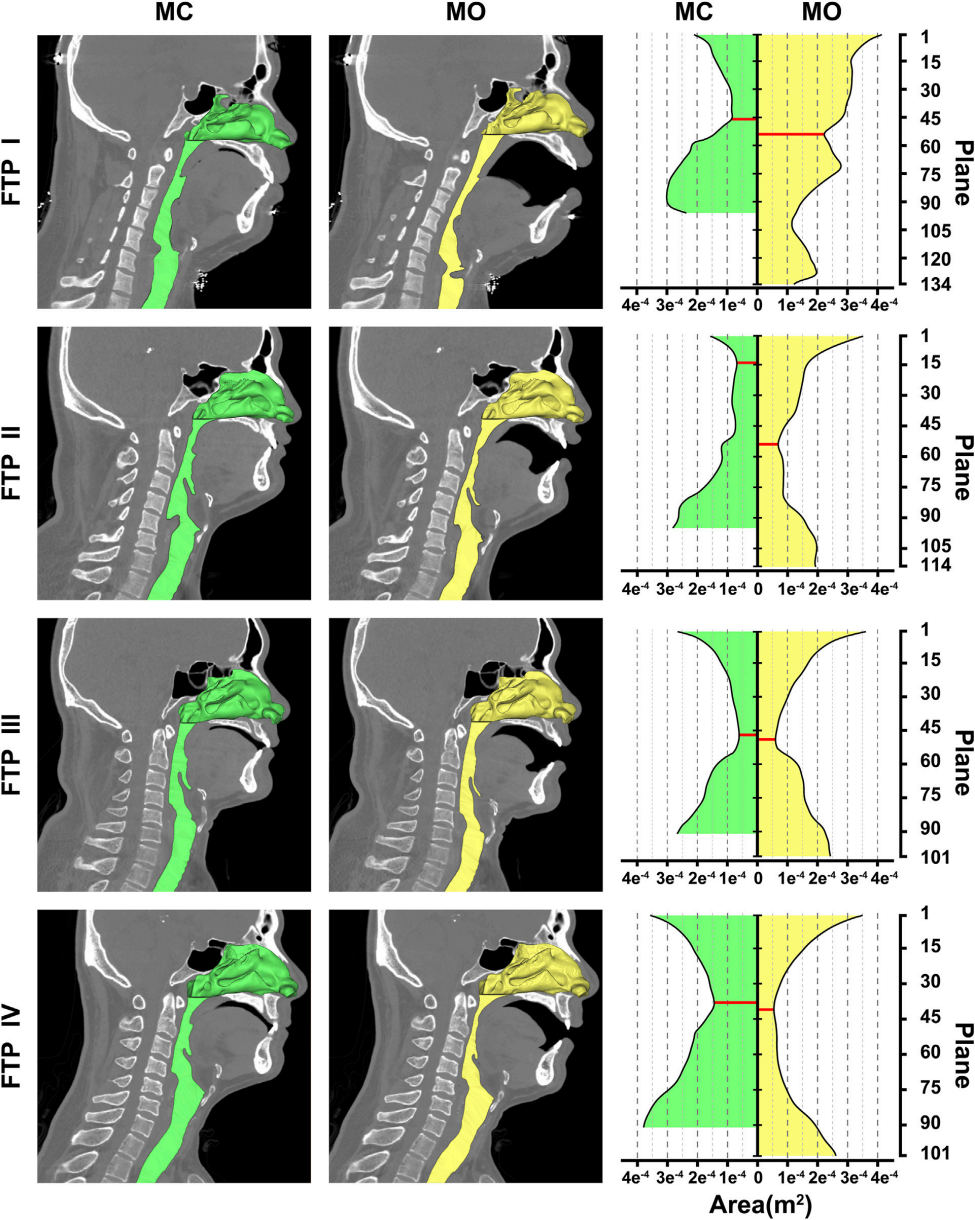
Morphological changes of the airway between MC and MO, Note, the airway models were embedded on the median sagittal CT image in the left two columns. The nasal cavity and nasopharynx presented in the form of stereoscopic (three-dimensional, 3D) and the remaining airway presented with sagittal profile. The green color represented the baseline airway lumen and the yellow color represented the airway lumen after MO. The area contours of cross-sectional planes from the level of the hard palate to the tip of epiglottis were showed in the right column. The horizontal axis represented the area of planes (m^2^); the longitudinal axis represented the plane N. The red solid line represented the plane_Amin_. (MC, mouth closed position; MO, mouth opening position; FTP, Friedman Tongue Position).
